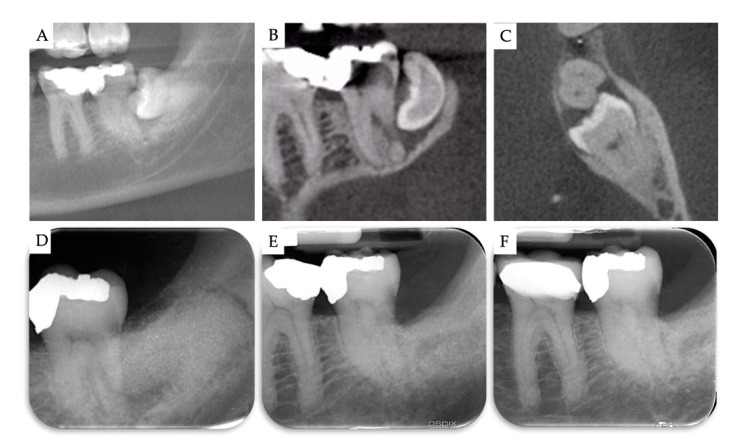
Case no 3. (A–C) CBCT images at T0. Periapical X-rays (D) immediately after the removal of M3 and grafting with β-TCP/CS, (E) 10 months post-operatively, and (F) 1 year post-operatively.
